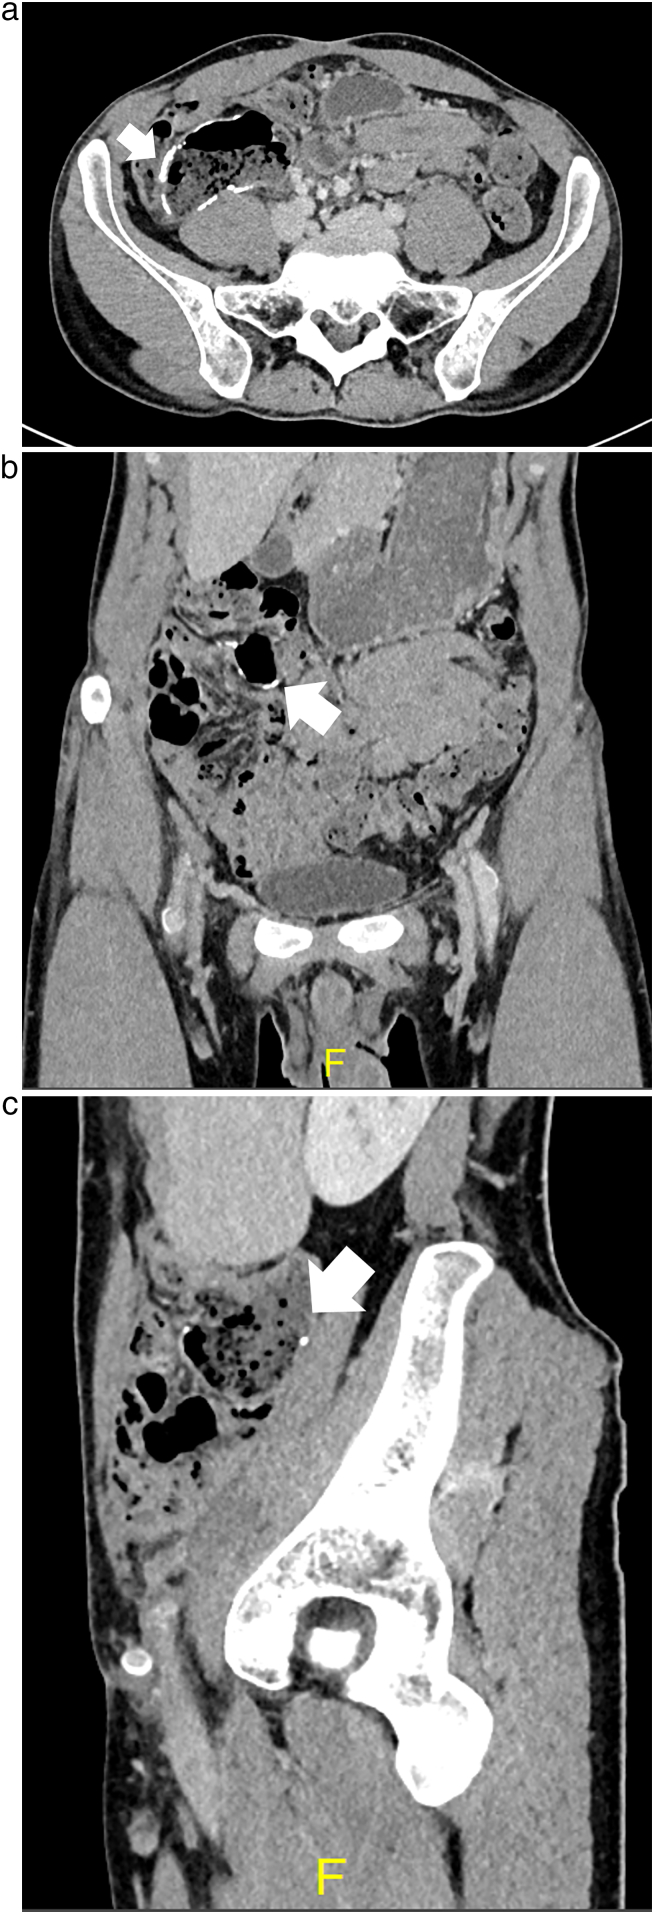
(a) Computed tomography (CT) findings following resection (axial). Anastomosis (arrow). (b) CT findings following resection (coronal). Anastomosis (arrow). (c) CT findings following resection (sagittal). Anastomosis (arrow).
